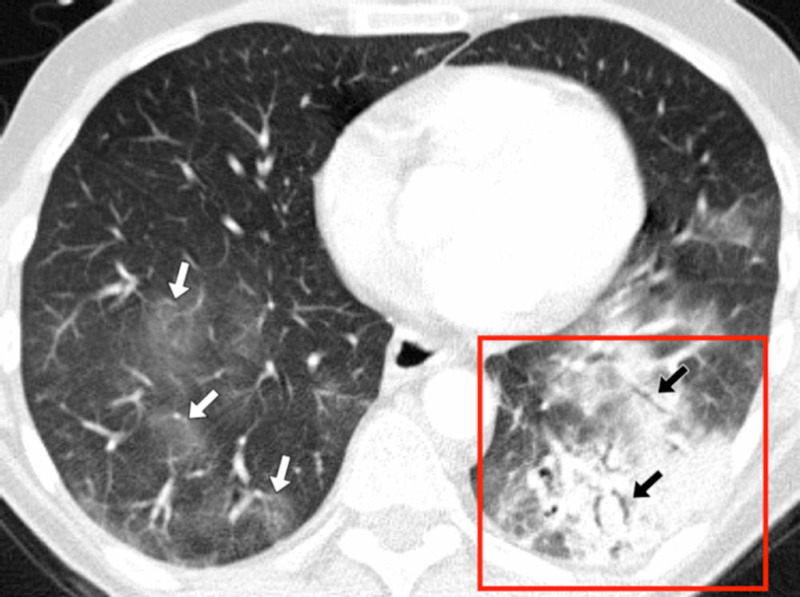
Patient 4 A 51-year-old male patient was admitted to the emergency department with a two-day history of vomiting. His abdomen was distended, with palpable bowel loops. He was investigated with whole-body computed tomography (CT). His abdominal CT (not shown) demonstrated small bowel obstruction, and imaging of the chest showed evidence of parenchymal changes consistent with COVID-19. Figure 4 shows an axial contrast-enhanced CT image of the chest, in lung windows. The image shows left basal consolidation (red box) with air bronchograms (white arrows). There are multiple, right, lower lobe ground glass-opacities (black arrows).
